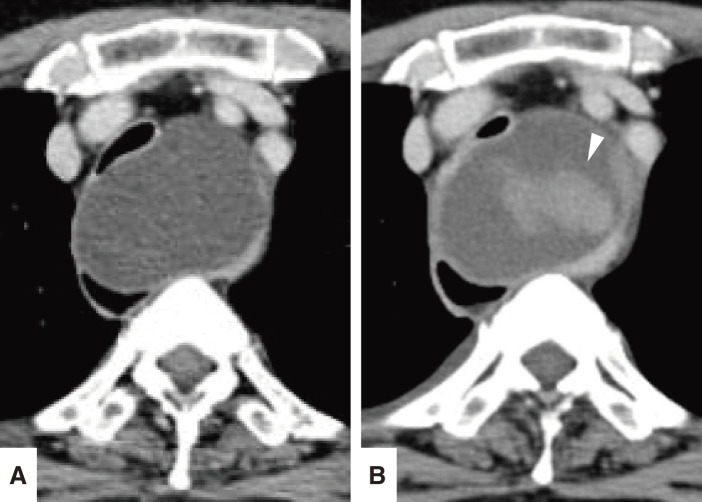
(A) Initial contrast-enhanced CT image showing a simple mediastinal cyst between the trachea and the esophagus. (B) Contrast-enhanced CT 3 days after the EUS-FNA showing contrast enhancement within the mass, suggesting an intracystic hemorrhage (arrowhead). EUS-FNA, endoscopic ultrasound-guided fine-needle aspiration
